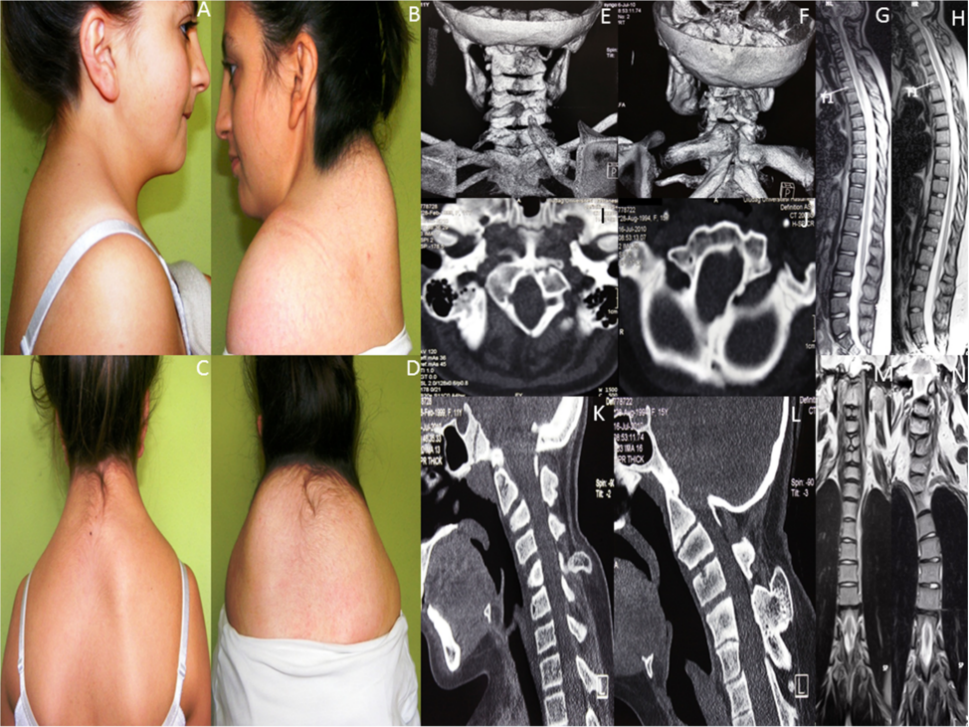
Patient photographs of family members IV-2 and IV-3 show KFS phenotypes. Photographs of patients from the side (A and B) and behind (C and D) show short neck, low posterior hairline, and elevated scapula (Sprengel’s deformity). 3D cervical spine skull base CT images show omovertebral bone causing Sprengel’s deformity, posterior neural arch fusion defects, and fusions between vertebrae (E and F). Whole spine sagittal section T2-weighted MR images show cervical vertebrae count anomaly (G and H). Axial skull base CT images show malformation of the foramen magnum (I and J). Sagittal reconstruction of cervical and skull base CT images show abnormal bony formation emerging from posterior structures as well as fusion between posterior structures of some successive vertebrae, occipitalization of atlas and dens, and corpus anomalies (K and L). Scoliosis is evident on coronal T2-weighted whole spine MR images. Fusion defects are seen in midportions of vertebra corpuses (M and N).
